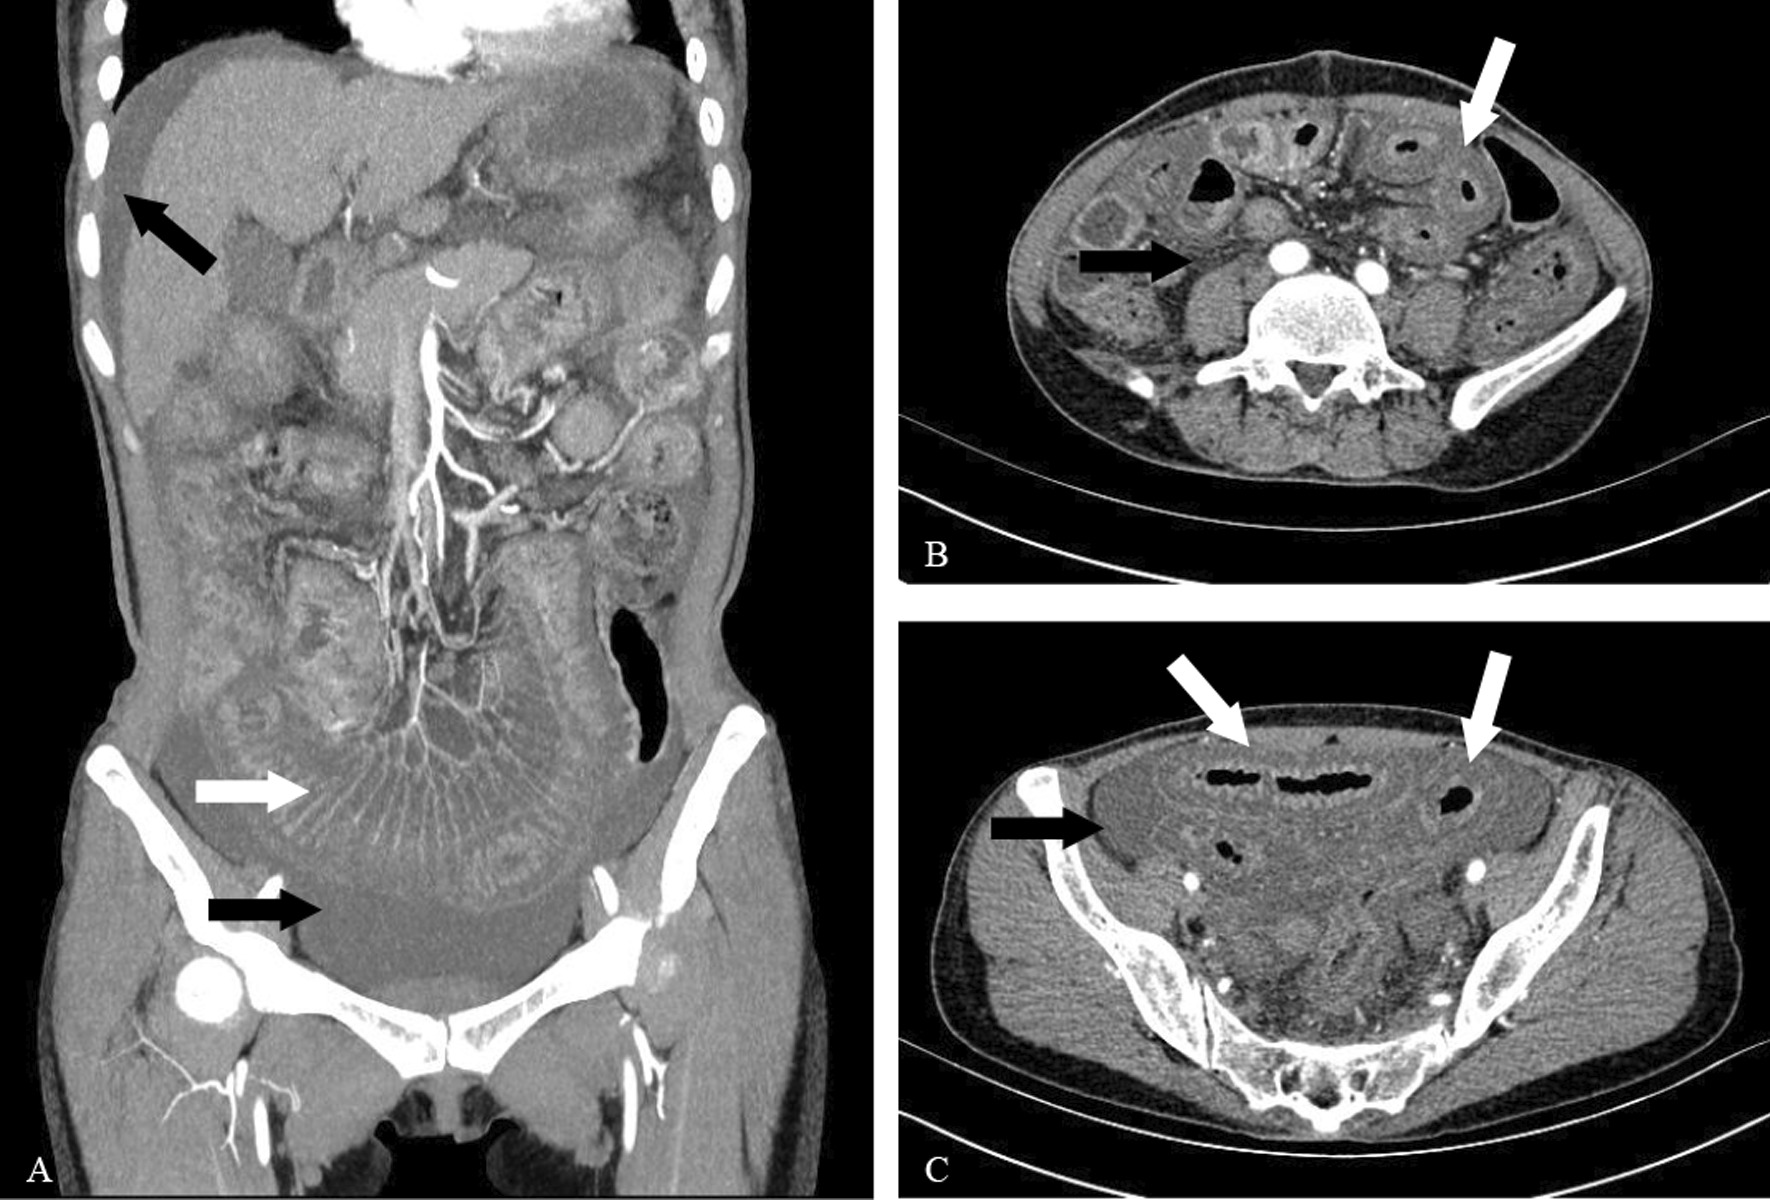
The CT manifestation of lupus enteritis. A: massive fluid in the abdominal cavity (the black arrow) and engorgement of mesenteric vessels (comb sign, the white arrow); B: increased attenuation of mesenteric fat (the black arrow) and the bowel wall thickening (target sign, the white arrow); C: massive fluid in the abdominal cavity (the black arrow), the bowel wall thickening and bowel dilatation (the white arrow)
